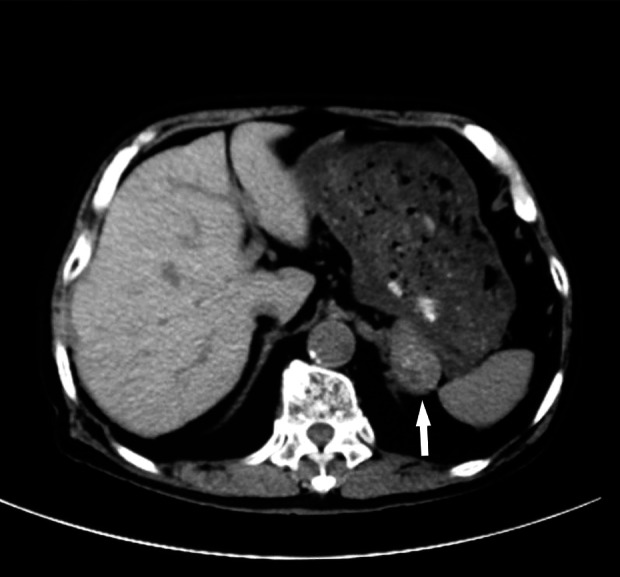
Post-contrast CT screen obtained four months previous to admission showing a homogeneous tumor (arrow) measuring ∼4×4 cm, which invaded the stomach wall. CT, computed tomography.
